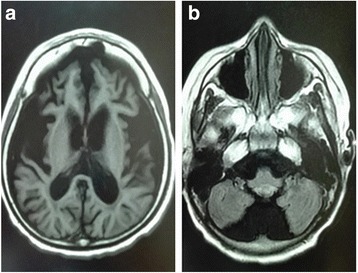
T1-weighted MRI images for the patient showed cerebral (a) and cerebellar (b) atrophy
